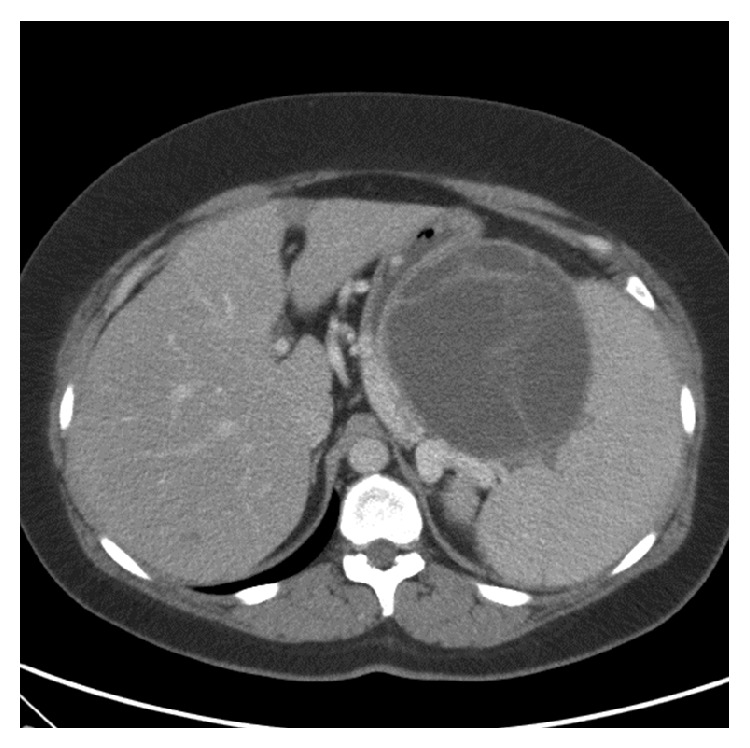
A computed tomography scan of the abdomen. Large cystic tumor in the tail of the pancreas along with moderate splenomegaly.
